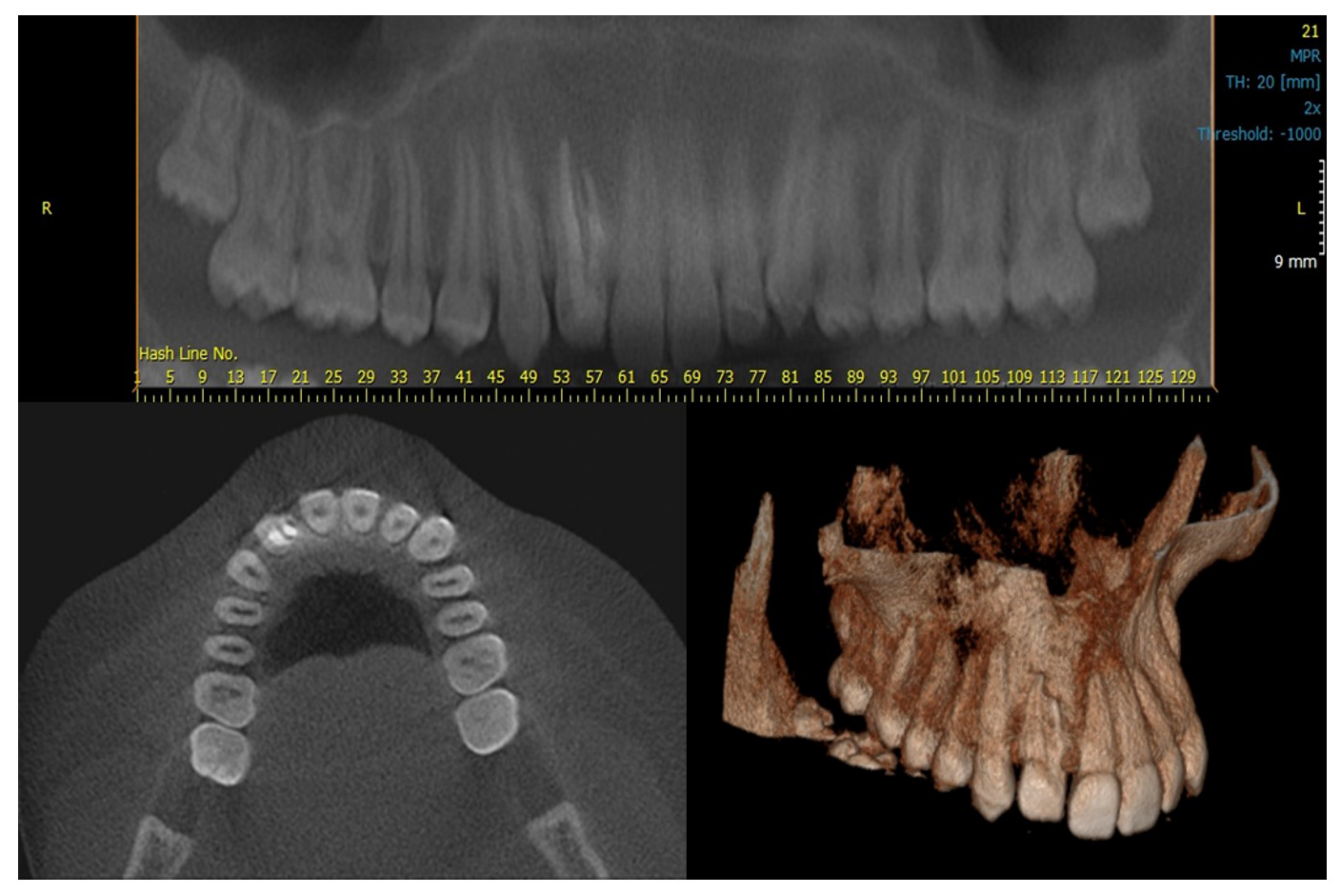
Cone beam computed tomography images 6-years after the root canal treatment in case 1.
